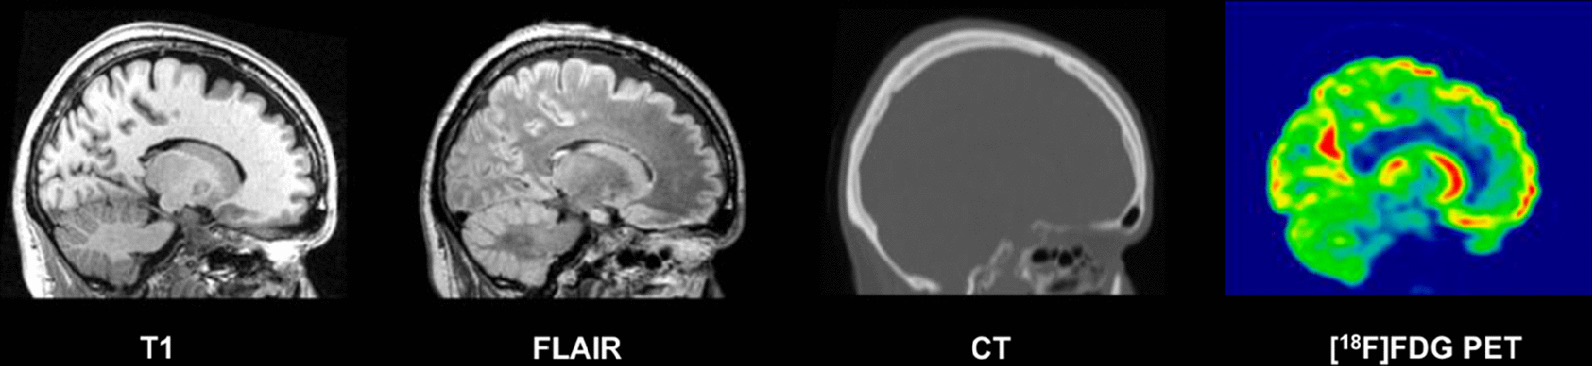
Example of normalized T1 MRI, FLAIR MRI, CT and [^18^F]FDG PET images (sagittal plane) in MNI space, for one subject of the database
